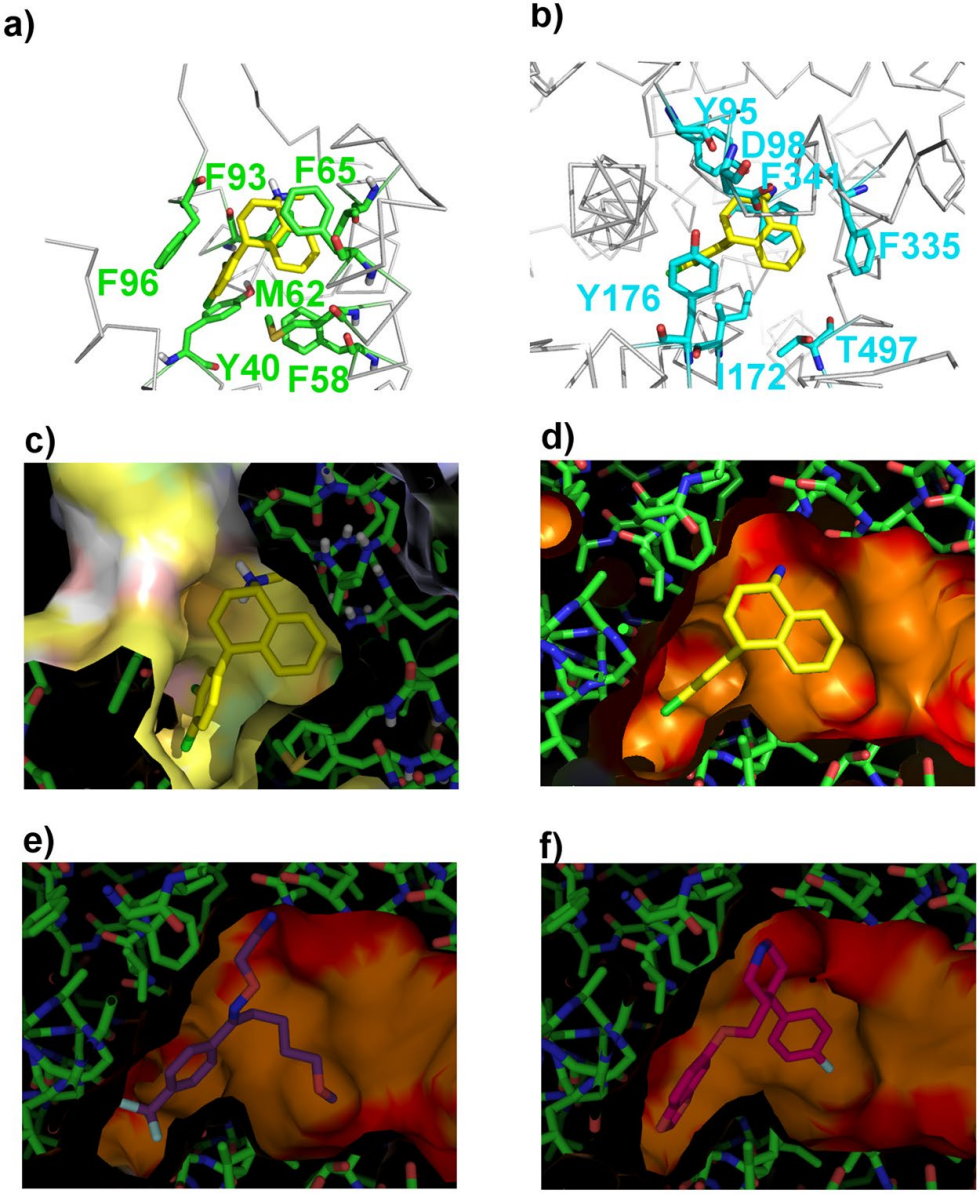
Structural comparison of the binding pockets of PAH1 and the serotonin transporter. (a) Docking structure of PAH1–sertraline represented as a ribbon model. Important residues in the interaction are represented as a stick model. Sertraline atoms are colored by type (yellow, carbon; white, hydrogen; blue, nitrogen; green, chlorine). (b) X-ray structure of the serotonin transporter (SERT)–sertraline complex (PDB code: 6AWO). Important residues in the interaction are represented as a stick model. Sertraline atoms are colored by type as in panel (a). (c) Binding site structure of the PAH1–sertraline docking calculation represented as a surface model using eF-surf ^78^, colored by default. (d–f) Binding site structures of 6AWO, 6AWP and 6AWN, respectively, represented as a surface model using eF-surf, colored by default.
